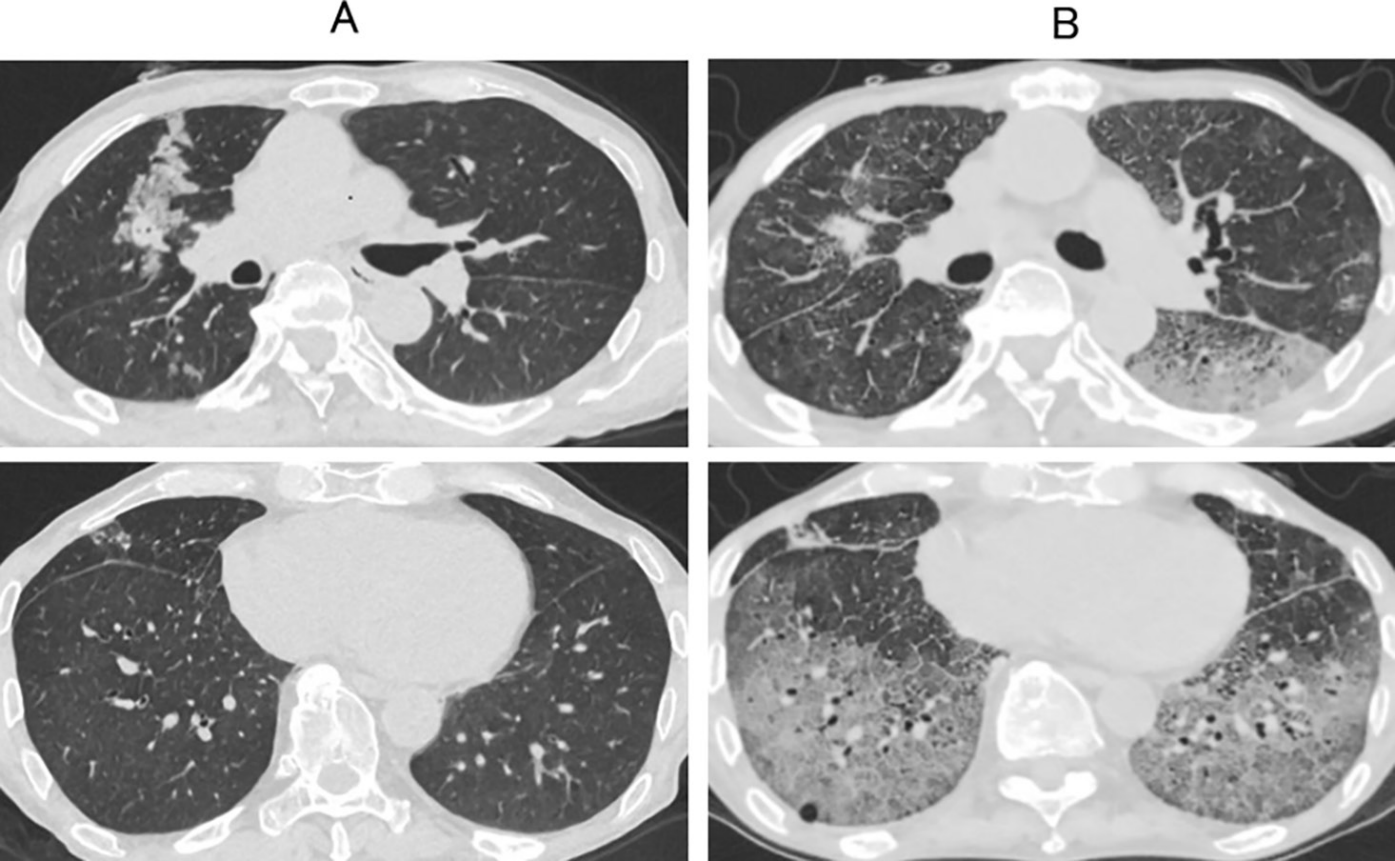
(A) Chest CT scan before pembrolizumab initiation. (B) Chest CT scan at the time of hospitalisation.
